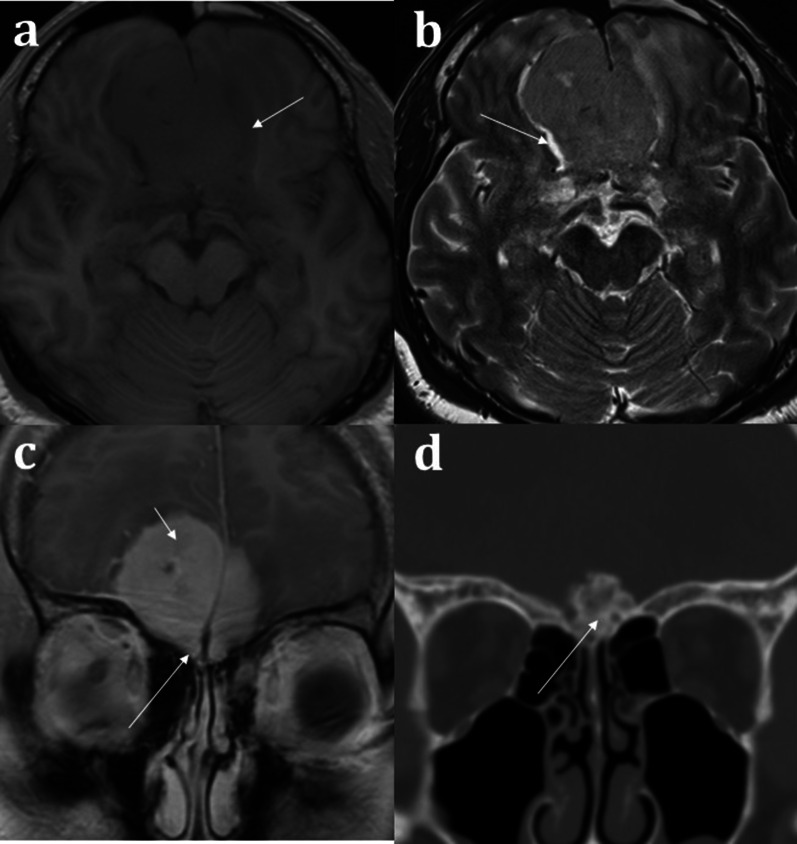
Olfactory groove meningioma. 51-year-old male presenting with headache. A large central intermediate intensity lesion is seen on T1W (a) and T2W imaging (b). It occupies the anterior skull base centrally crossing the midline and demonstrates grey matter immediately adjacent to the lesion (arrow, a) and a CSF cleft (arrow, b) indicative of an extra-axial location. The lesion demonstrates homogenous intense enhancement following contrast administration (short arrow, c). The coronal bone window CT (d) demonstrates hyperostosis of the underlying anterior cranial fossa (long arrows), typical for a meningioma. Despite the marked mass effect on the olfactory sulcus and intimate association with the olfactory groove (long arrow, c), the patient only complained of a mild olfactory disturbance
